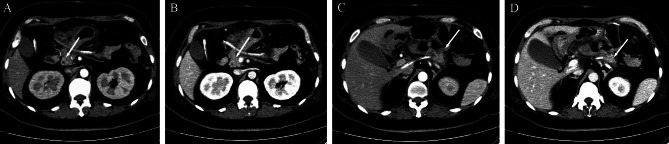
Postoperative CT images after Roux-en-Y Pancreaticojejunostomy. A: Arterial phase of contrast-enhanced CT; B: Venous phase of contrast-enhanced CT; C: Arterial phase of contrast-enhanced CT; D: Venous phase of contrast-enhanced CT; Note: The arrows in pictures A and B indicate the residual pancreatic head; The arrow in picture C indicates the location of the anastomosis of pancreaticojejunostomy; The arrow in picture D indicates the residual pancreatic tail
